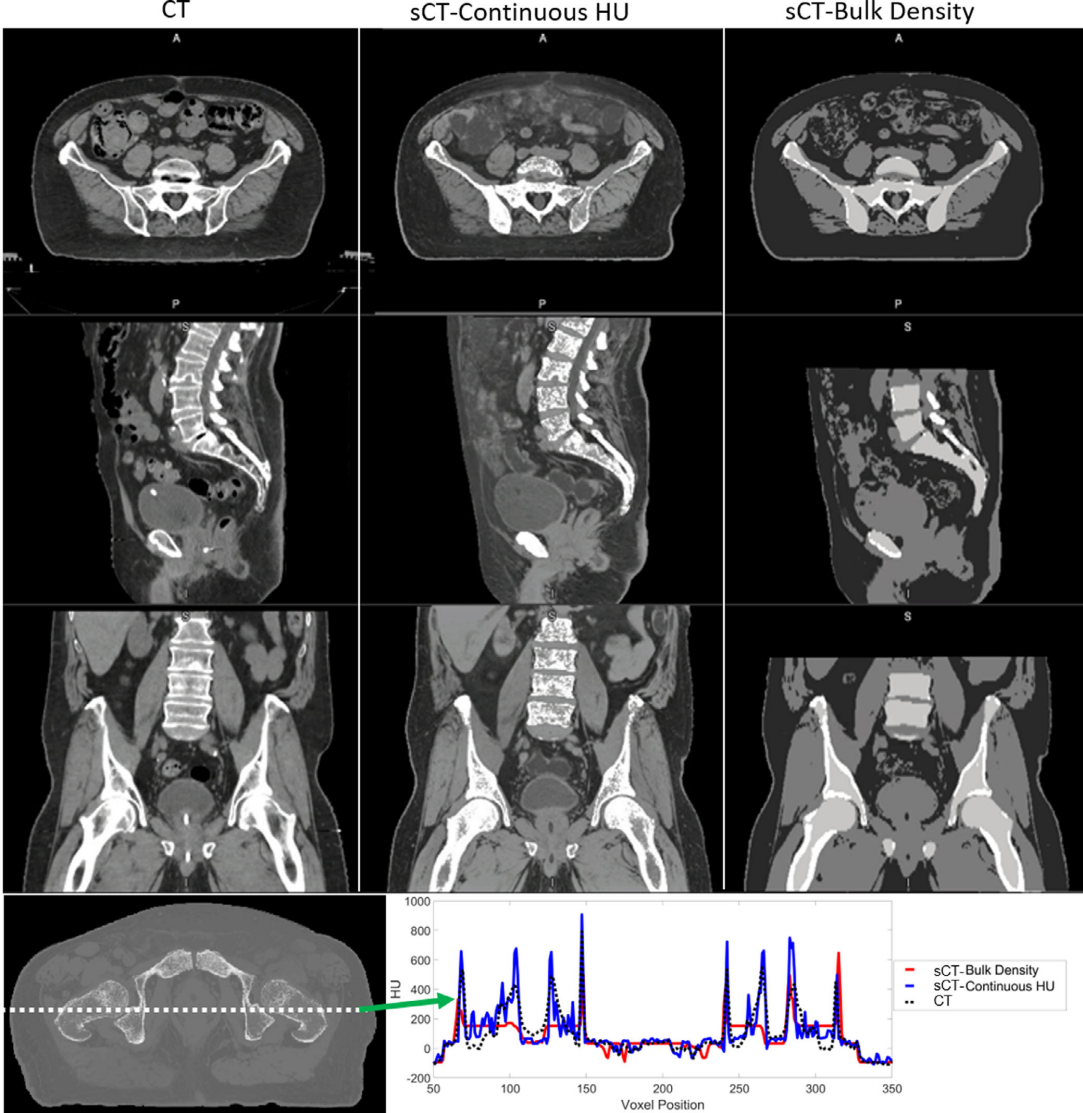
An example case comparing planning CT, continuous HU synthetic CT and the bulk density synthetic CT. HU profile comparison between CT and the continuous HU and bulk density synthetic CTs. CT: black dashed line, continuous HU synthetic CT: blue, bulk density synthetic CT: red (CT: computed tomography, HU: Hounsfield unit, A: anterior; P: posterior, S: superior, I: inferior).
